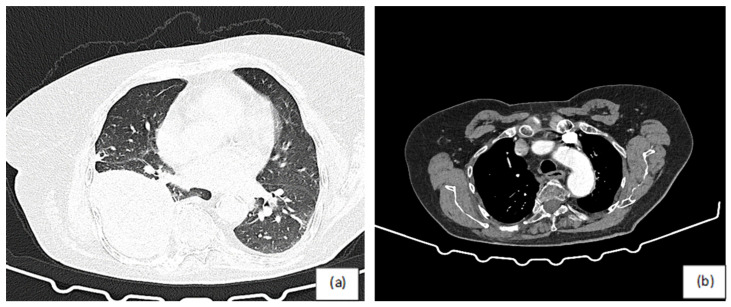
Chest CT at the current admission. (a) Pleura-adherent tumoral mass with smooth margins, located in the right lower lobe. (b) No pleural effusion or mediastinal lymphadenopathy. Source: authors’ archive.
